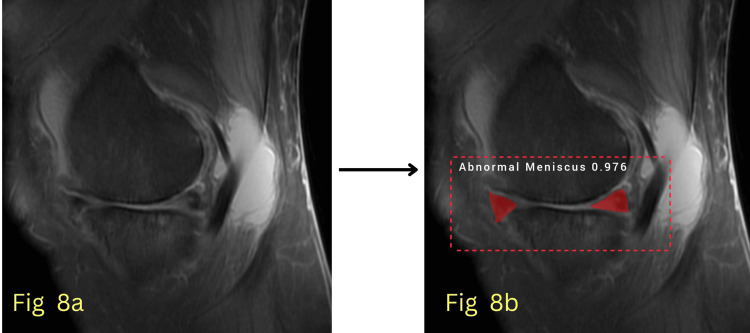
(a) Input MRI image; (b) Segmented image showing abnormal anterior and posterior horns of the meniscus.
